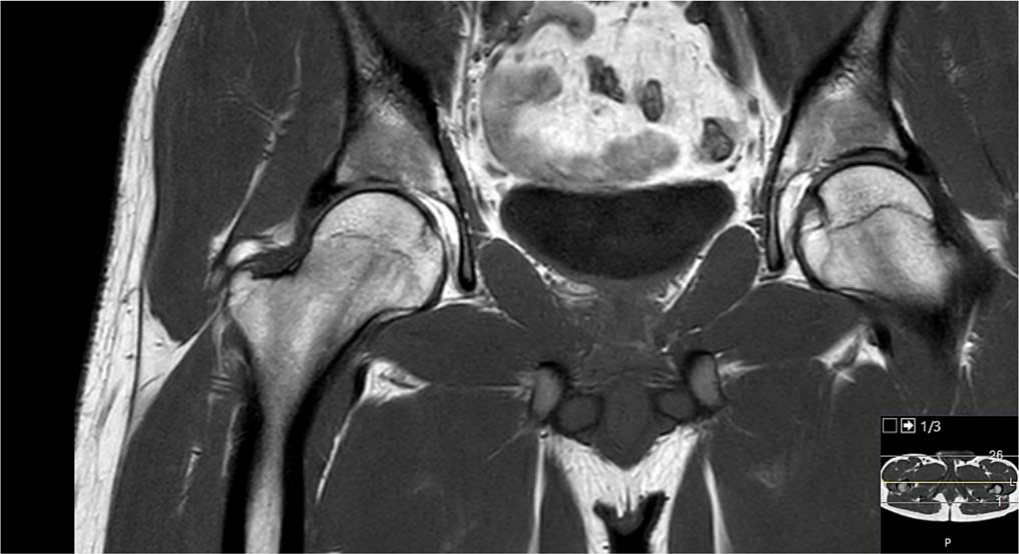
MRI of the same patient with ossified labrum.
